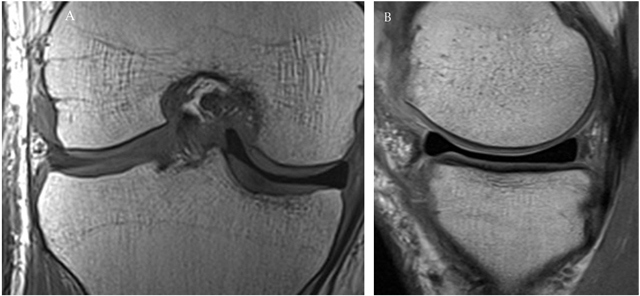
Total medial meniscus implant. Coronal (A) and sagittal (B) T1-weighted fast spin echo MR-images demonstrating the discoid medial meniscus implant with homogenous low signal intensity.
